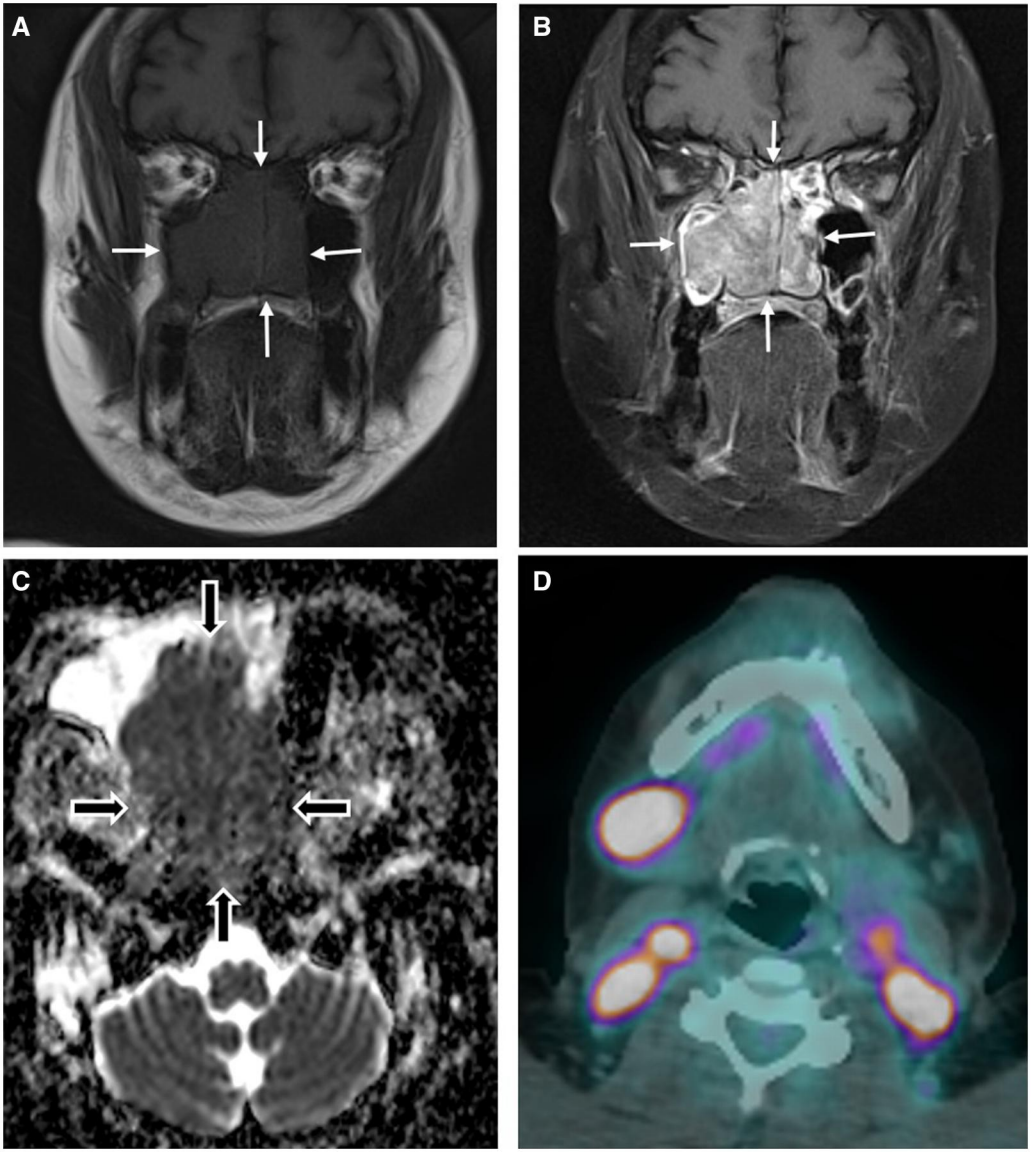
Extranodal NK T-cell lymphoma of the sinonasal system. (A) Coronal non-fat-suppressed T1-weighted and (B) fat-suppressed post-contrast T1-weighted MRI show a low-to-intermediate signal homogeneous nasal cavity and right maxillary antral lesion (white arrows) that enhances relatively homogeneously. (C) ADC map shows markedly low values (black arrows) visually similar to EMP and (D) FDG-PET/CT from the same patient demonstrates multiple avid right level 1b and bilateral level 2 cervical lymphadenopathy which is a useful discriminator from plasma cell neoplasms.
